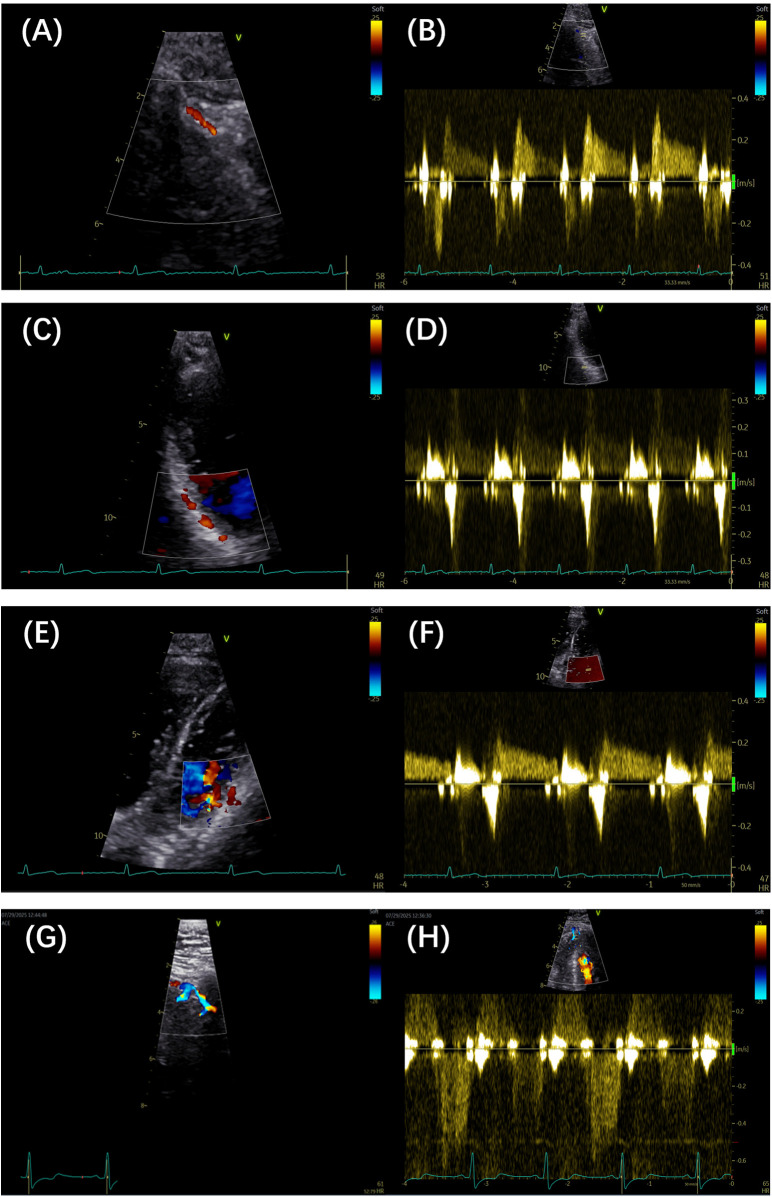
(A,B) Color Doppler and pulse Doppler detection of the LAD artery, respectively. (C,D) Color Doppler and pulse Doppler detection of the PDA, respectively. (E,F) Color Doppler and pulse Doppler detection of the LCX branch, respectively. (G,H) Color Doppler and pulse Doppler detection of septal branches, respectively. LAD, left anterior descending; PDA, posterior descending artery; LCX, left circumflex artery.
